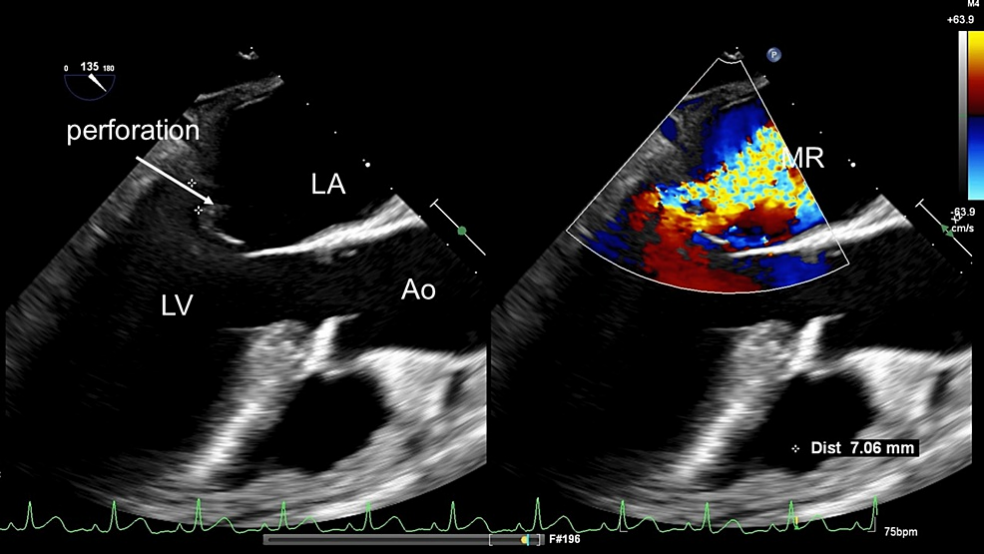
Preoperative TEE Preoperative TEE showed perforation (✜) of the PML TEE: Transesophageal echocardiography; MR: Mitral regurgitation; LV: Left ventricle; LA: Left atrium; Ao; Aorta; PML: Posterior mitral leaflet
